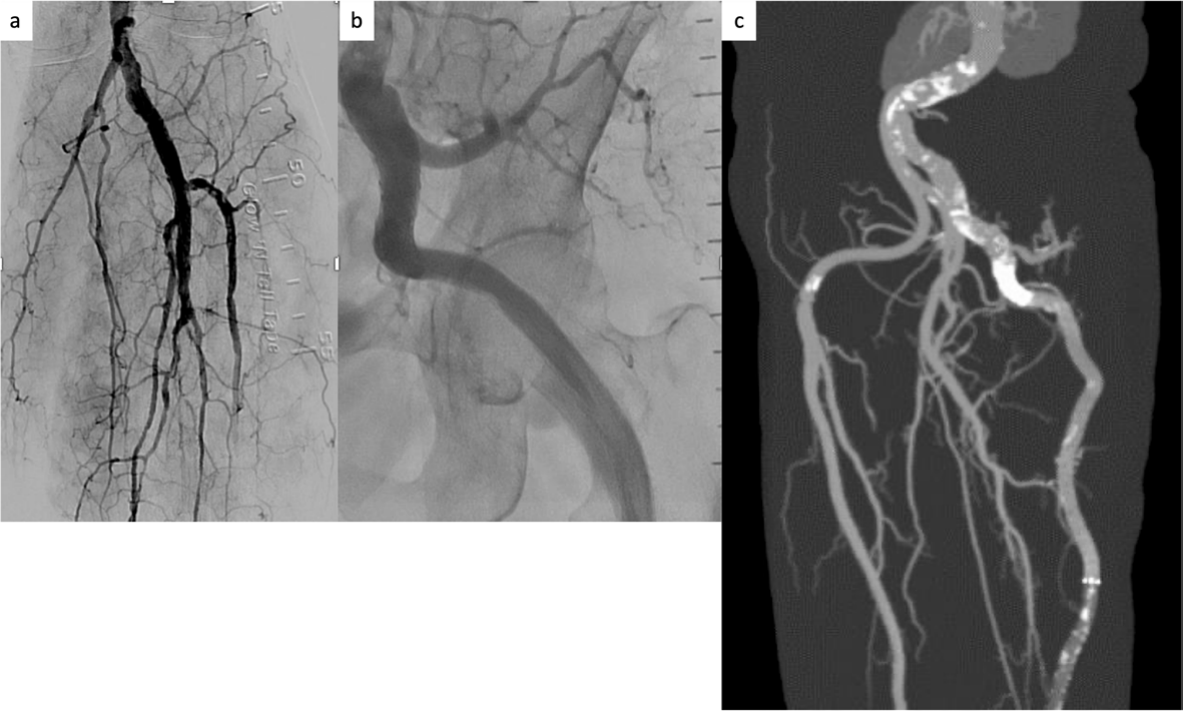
a, b Angiography revealing disappearance of the PSA aneurysms and two-vessel runoff. c Enhanced computed tomography angiography confirming disappearance of the PSA aneurysms. PSA, persistent sciatic artery
